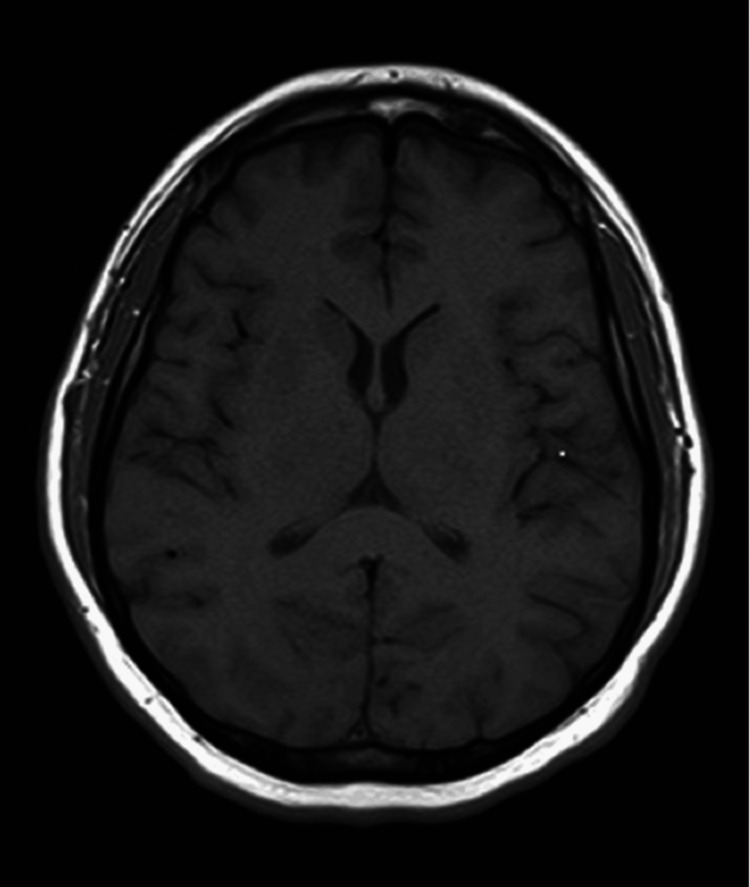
MRI of the brain showing no evidence of metastases
